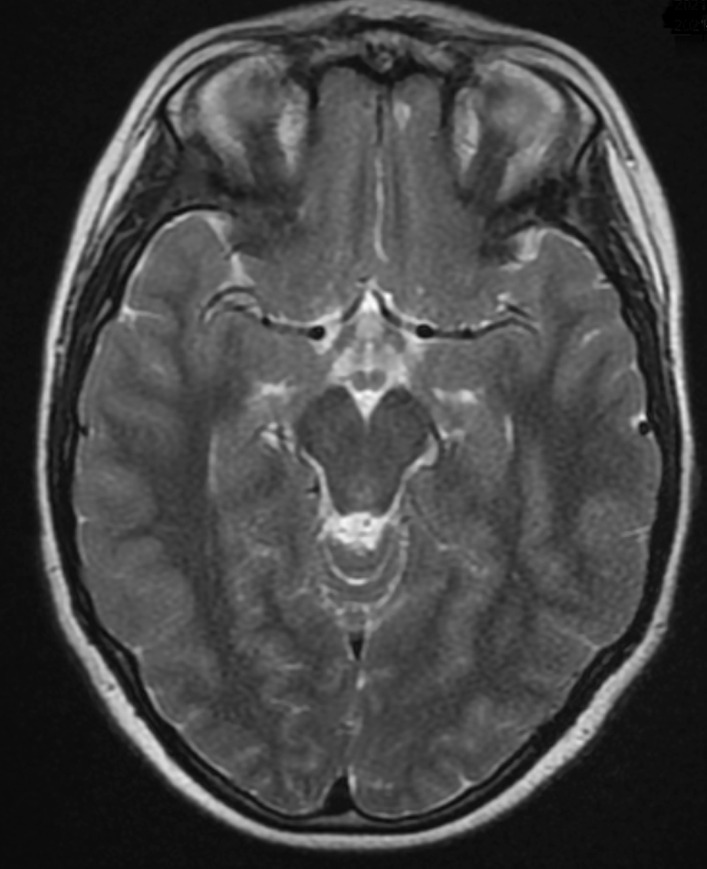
Image of brain MRI scan, Ax T2 FSE. Image of a brain MRI scan performed 3 months after the acute phase. Brain cortex hyperintense signal in both hippocampi and at the base of the right frontal lobe have resolved
